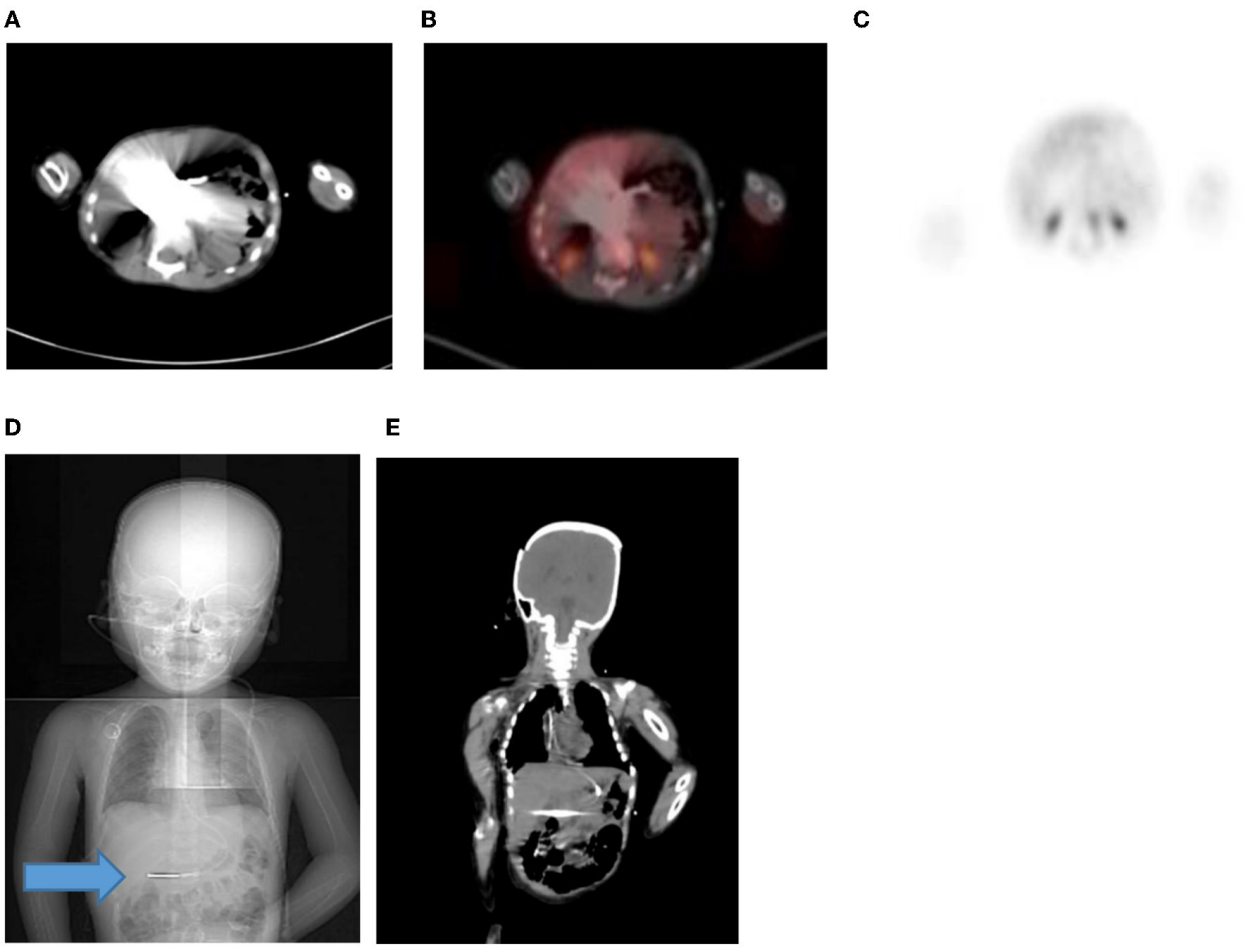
(A–C) 2 year old male with neuroblastoma who had a staging PET/CT. Artifact in the abdomen due to metal component of nasogastric tube *in-situ* resulting in streak artifact on CT (A) and fused PET/CT (B). Note the PET only image shows that there is normal excretion in the renal calyces with physiological low grade uptake in the liver. Coronal CT scannogram (D) shows the tube extending into the abdomen (Blue arrow) with streak artifact on coronal CT (E).
